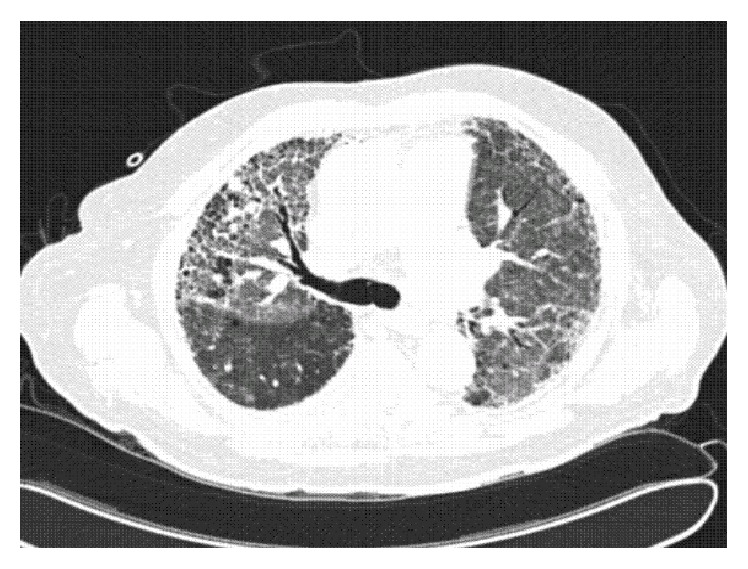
CT angiograph showing no evidence of acute pulmonary thromboembolism but the patient has extensive pulmonary fibrosis.
